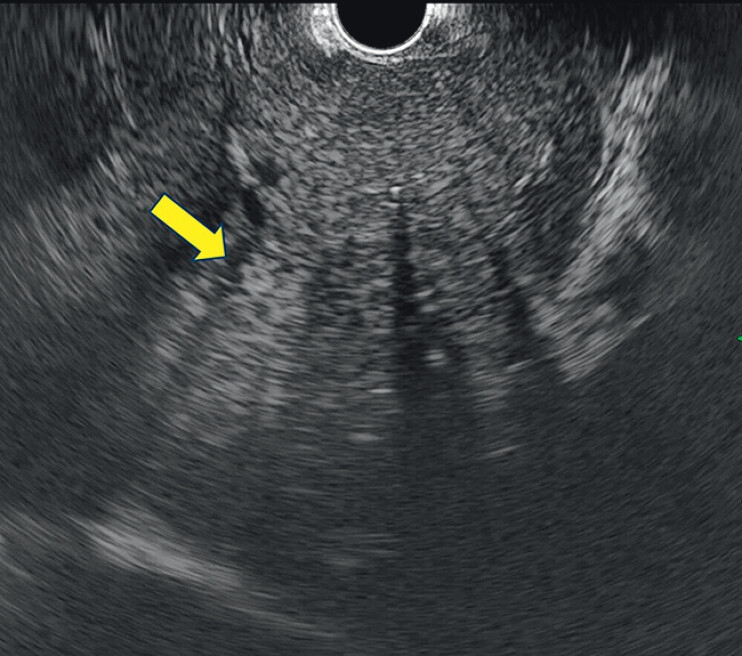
A hyperechoic lesion with a peripheral hypoechoic rim was detected in segment 4 of the liver (arrow). Endoscopic ultrasound-guided tissue acquisition was performed.
